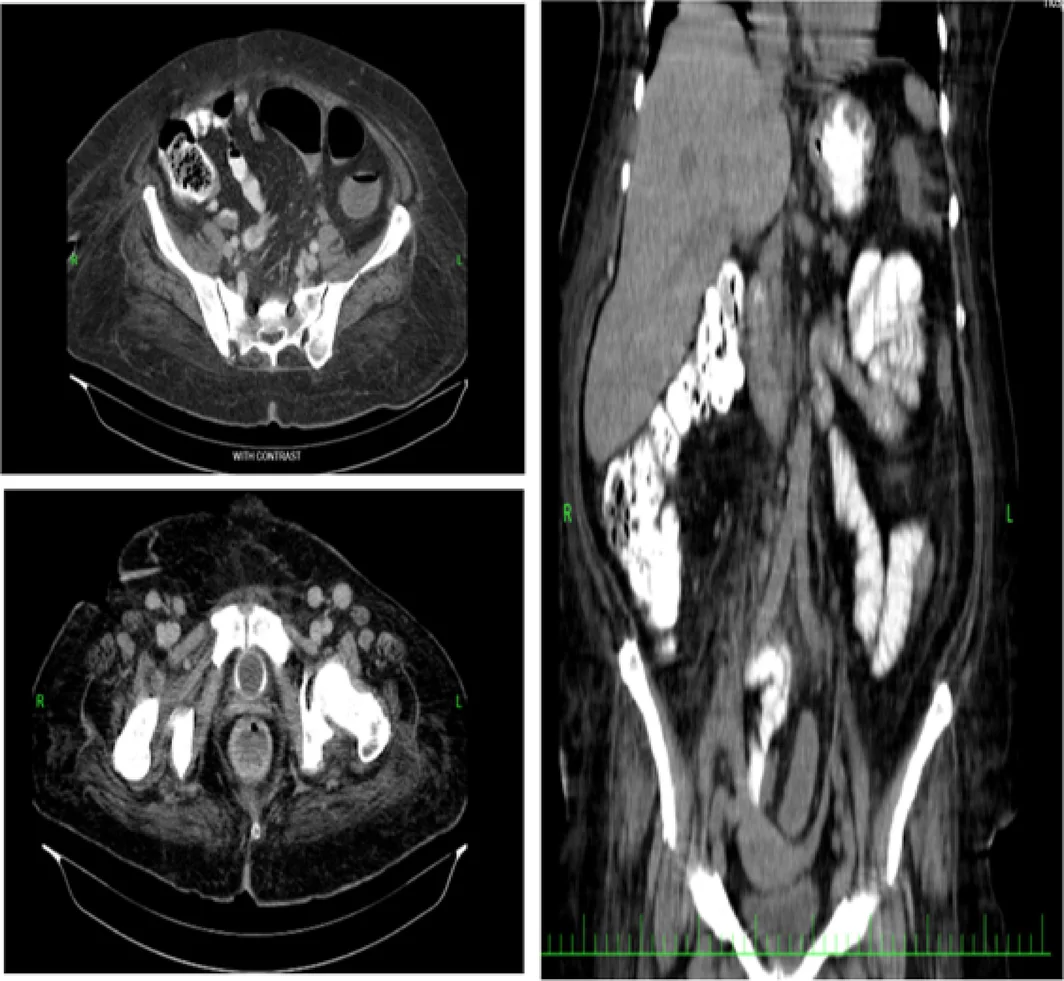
Axial and coronal contrast‐enhanced abdominopelvic CT demonstrating enlarged external iliac lymph nodes (arrows) with surrounding fat stranding, consistent with lymphadenopathy and possible inflammatory or infiltrative involvement.
